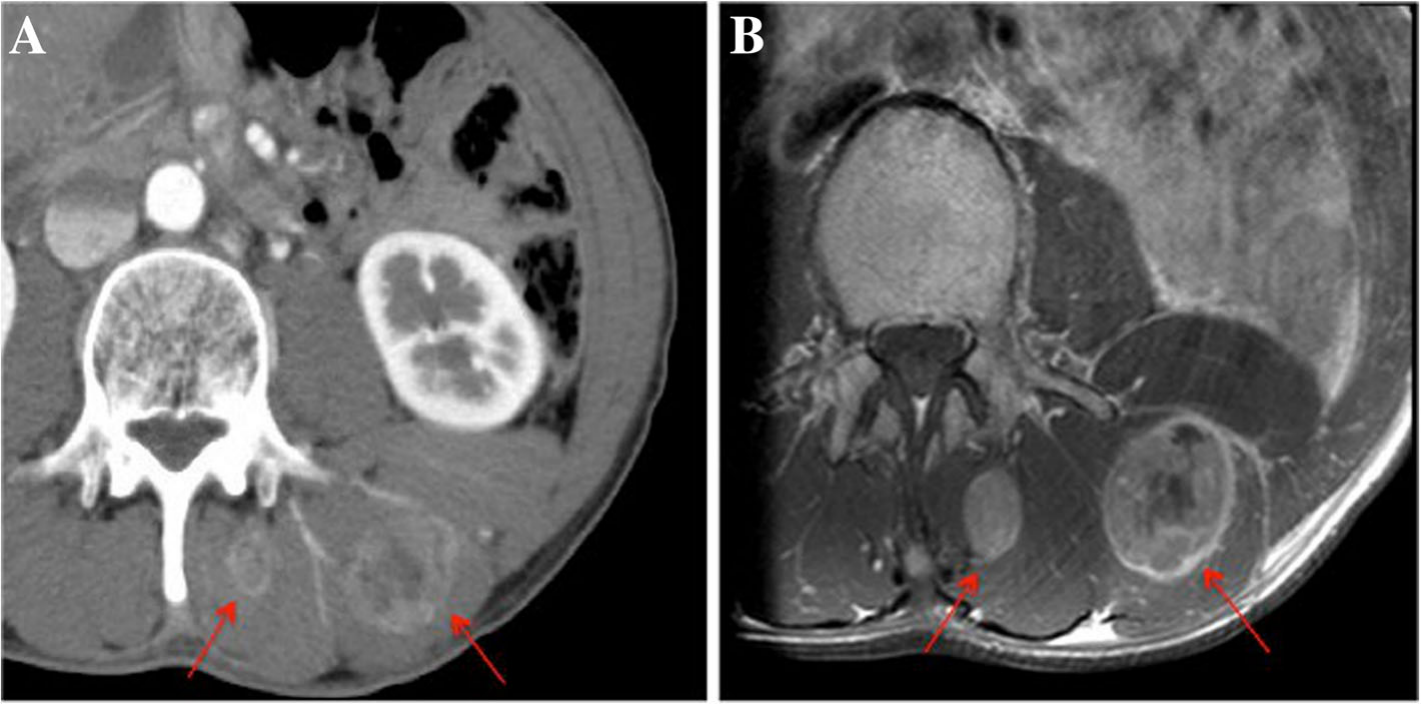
a An abdominal computed tomography scan revealed a solid mass (arrows), measuring 40 mm × 30 mm, in the left latissimus dorsi muscle. b A contrast-enhanced magnetic resonance imaging showed extensive peritumoral enhancement (arrows)
